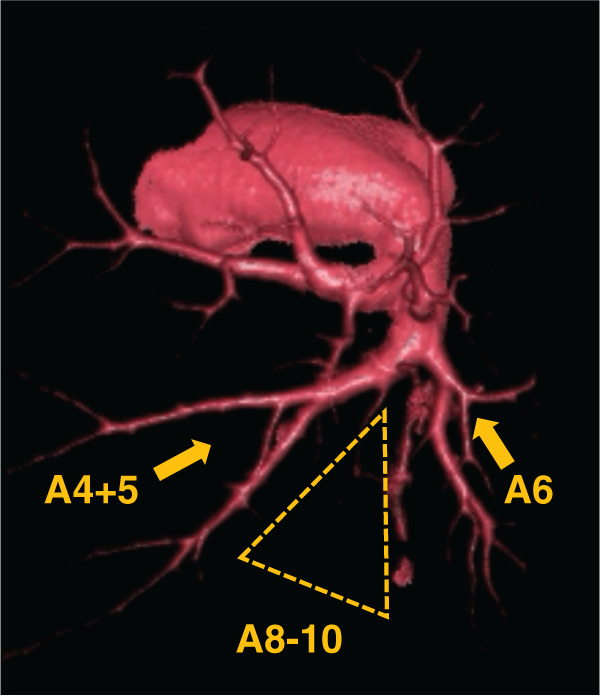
Preoperative 3D CT angiography of the left pulmonary artery. This figure demonstrates normal branches to A4+5 and A6 from the left pulmonary artery (arrows) without basal segmental branches (area enclosed by the dotted line).
